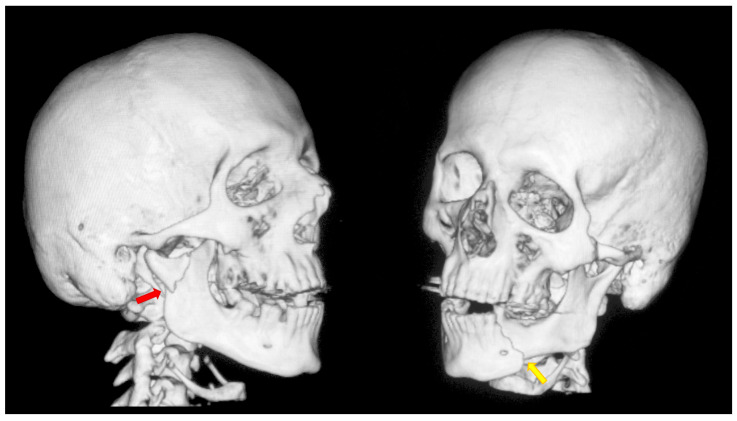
A typical case of mandibular injury. Computed tomography scan performed prior to treatment. The red arrow indicates a fracture at the base of the mandibular condyle. The yellow arrow indicates an additional fracture in the contralateral mandibular body. This is an epidemiologically typical situation [51]. A fracture of the mandibular condyle very frequently occurs together with another fracture of the mandible. The coexistence of two fracture lines causes significant instability of bone fragments and causes typical malocclusion.
